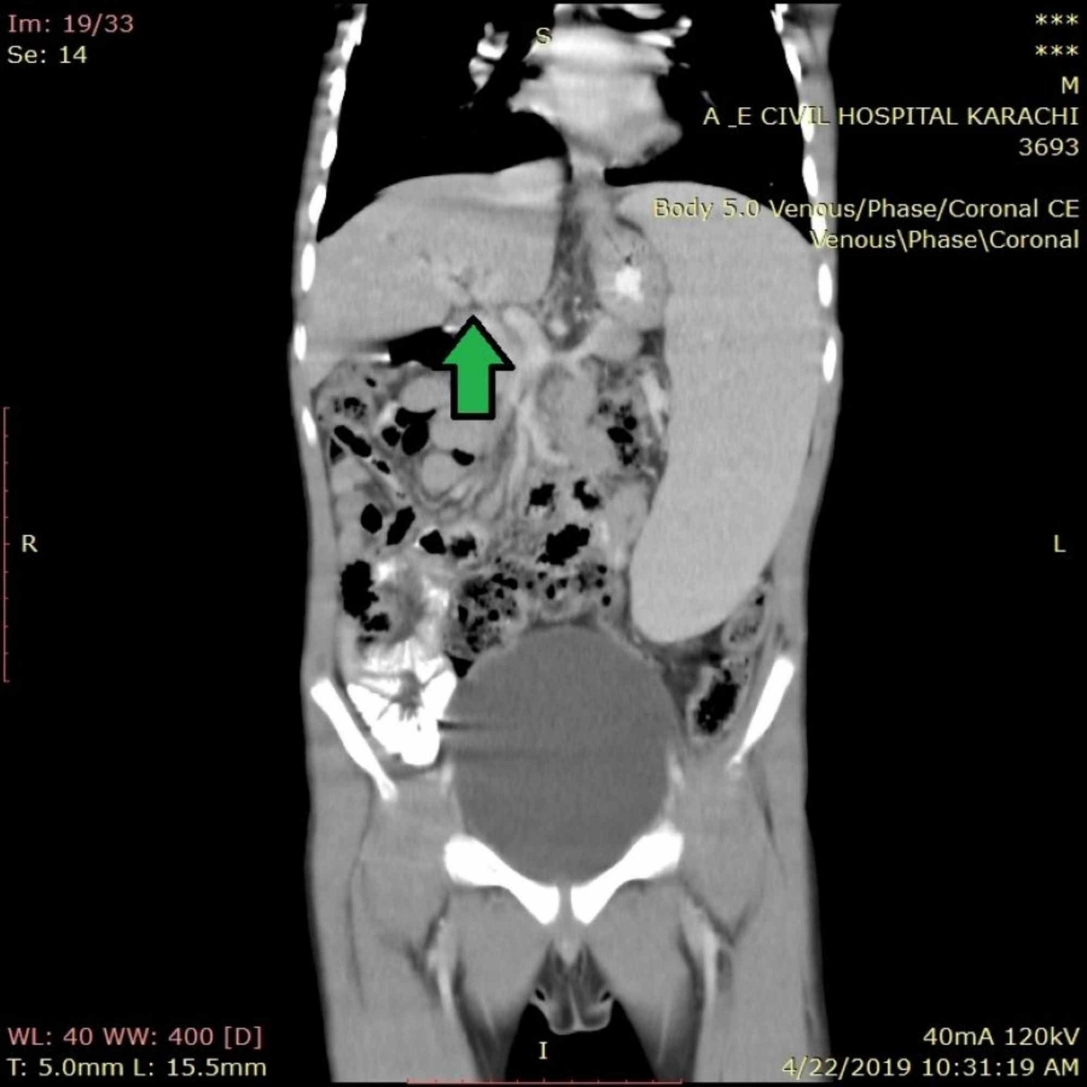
Coronal section of CT abdomen of patient showing cavernous transformation of portal vein at porta hepatis CT, computed tomography
